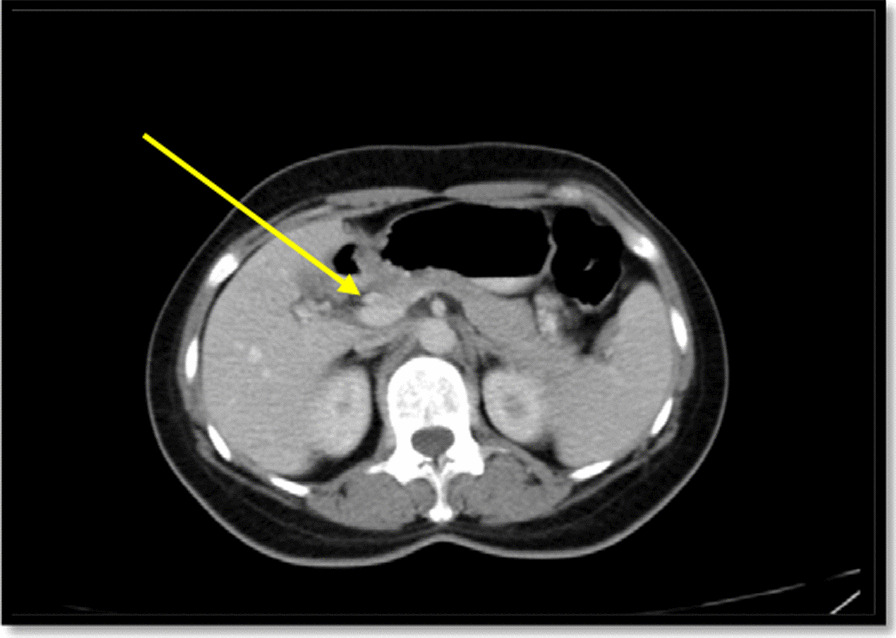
Spiral abdomen and pelvic CT scan: a lymph node within gastrohepatic ligament shown with a yellow arrow
